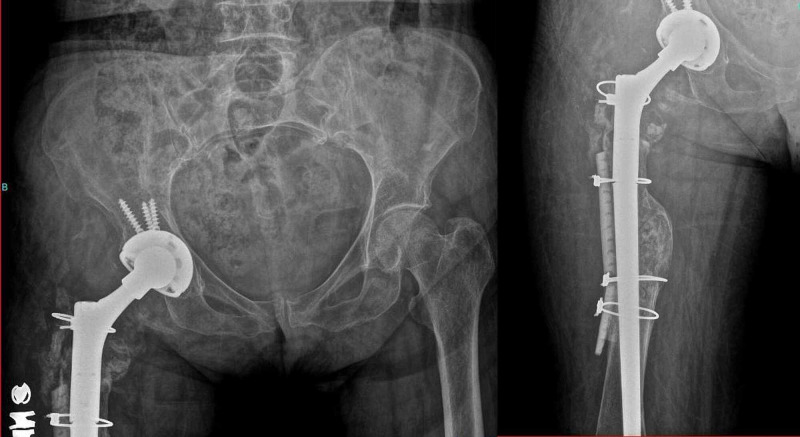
Postoperative one-year radiographs of the patient showing replaced femoral head, liner, and proximal modular part of femoral stem after revision due to the disassociation of the femoral head and neck components.
